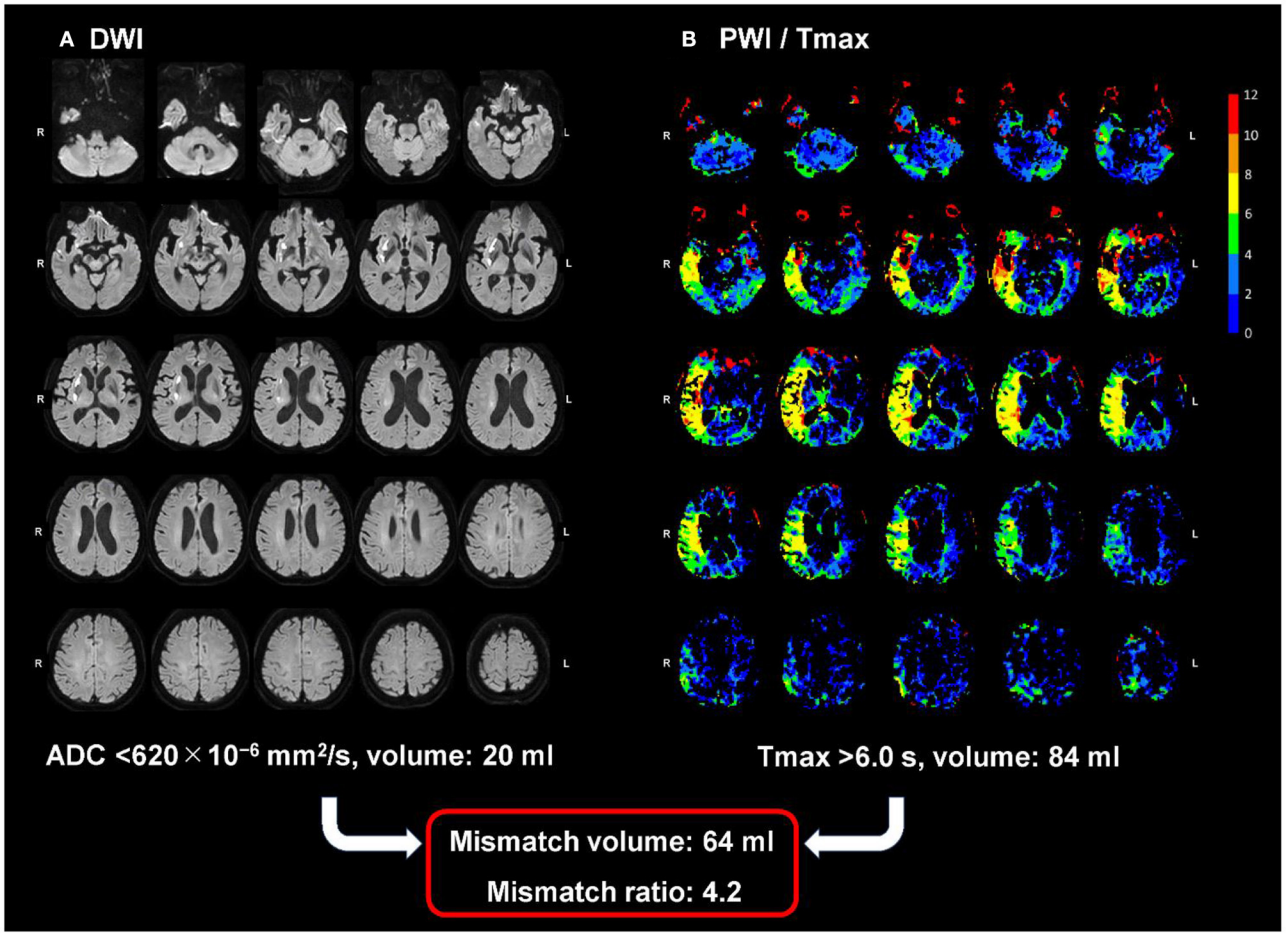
A representative case with Target Mismatch. The maps show DWI (A) and PWI/Tmax (B) with the right middle cerebral artery occlusion 90 min after symptom onset. The infarction core volume derived from DWI (apparent diffusion coefficient <620 × 10–6 mm^2^/s) is 20 ml and the ischemic penumbra volume derived from PWI (Tmax of >6 s) is 84 mL, indicating a mismatch ratio of 4.2 and a good candidate for reperfusion therapy. DWI, diffusion-weighted imaging; PWI, perfusion-weighted imaging.
